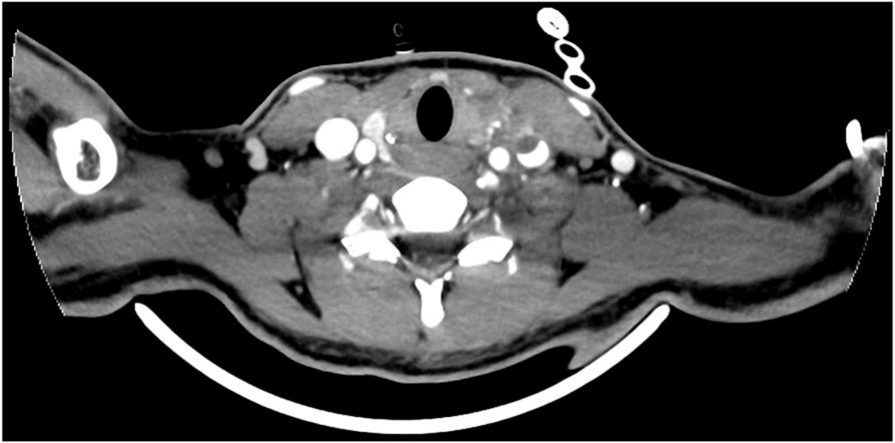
Cervical CT scan: partial filling of the left IJV, confirming thrombosis
